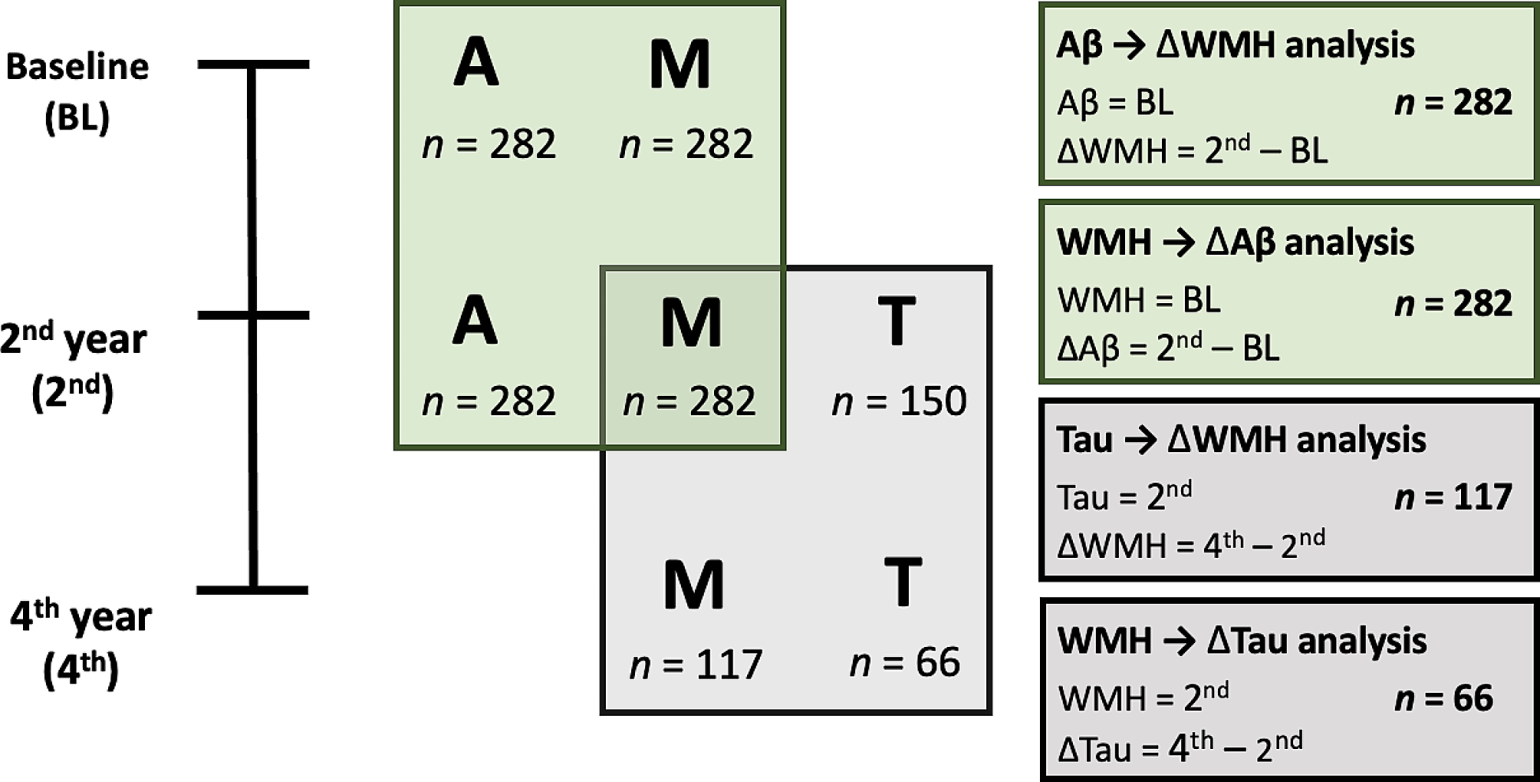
Datasets for analysis. Dataset for analysis of association between Aβ or Tau deposition and WMH volume. Aβ = Beta-amyloid, WMH = White matter hyperintensity. A = Aβ-PET, M = MRI scan, T = Tau-PET
